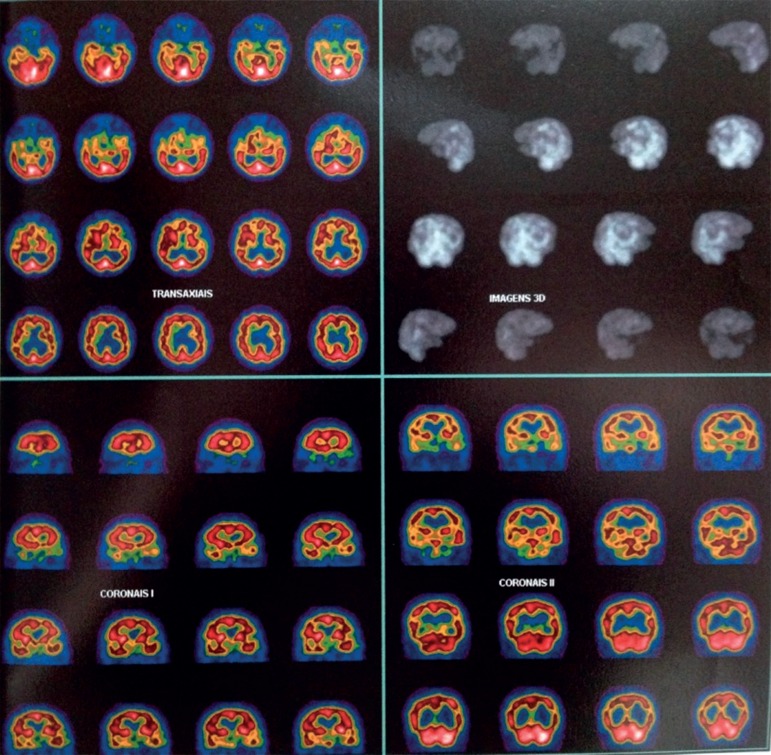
Single-Photon Emission Computed Tomography (SPECT). Image showing moderate-to-severe hypoperfusion/hypoactivation in regions
